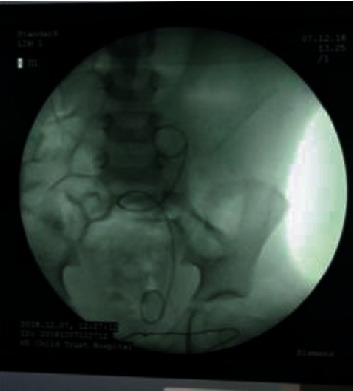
Xray KUB showing JJ stent across the left PUJ.
